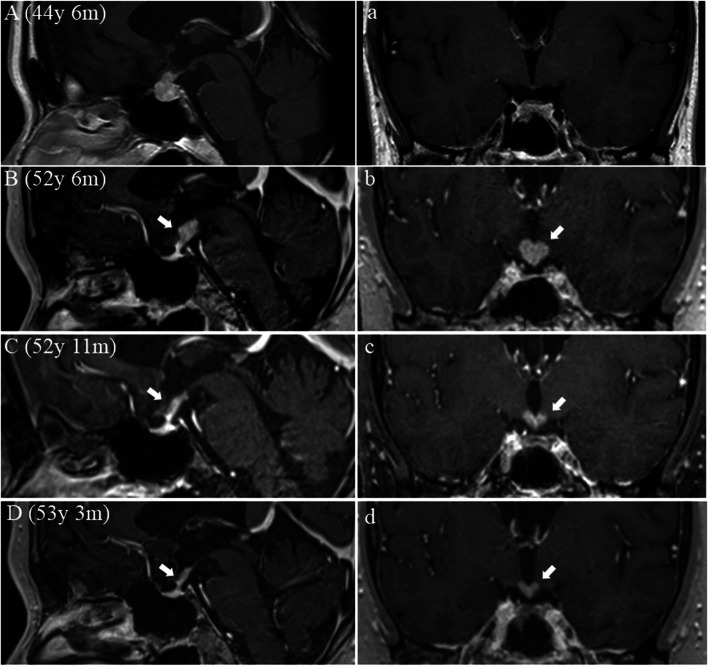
Head magnetic resonance imaging (sagittal, coronal, T1-weighted image with gadolinium). A, a When she developed central diabetes insipidus, hypertrophy with contrast-enhanced effects in both adenopituitary and neuropituitary and thickening of the pituitary stalk were seen. B, b When she developed memory impairment, contrast-enhanced effects in the pituitary stalk and the mammillary bodies of the hypothalamus were seen. After the first course of high-dose methylprednisolone pulse treatment (HDMPT) (C, c) and after the second course of HDMPT (D, d), the volume of the lesion was significantly reduced
